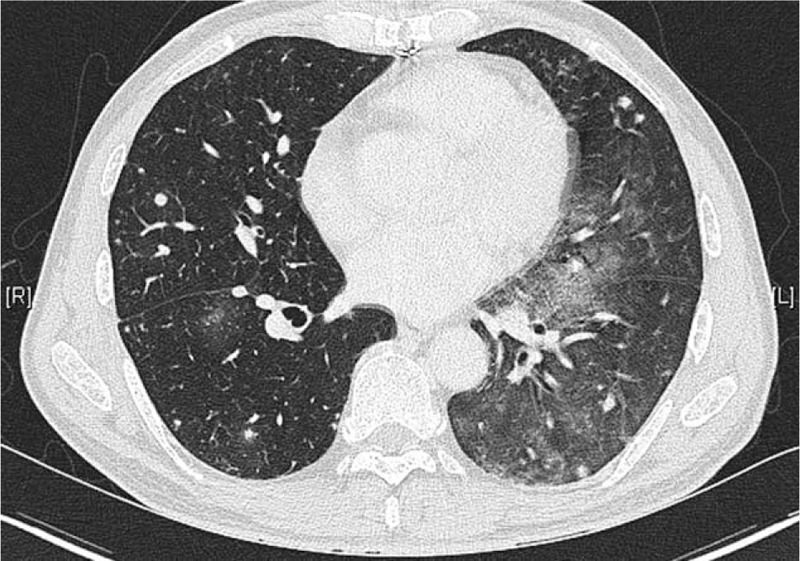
Chest computed tomography showing extensive metastatic lesions in the bilateral lung fields.
